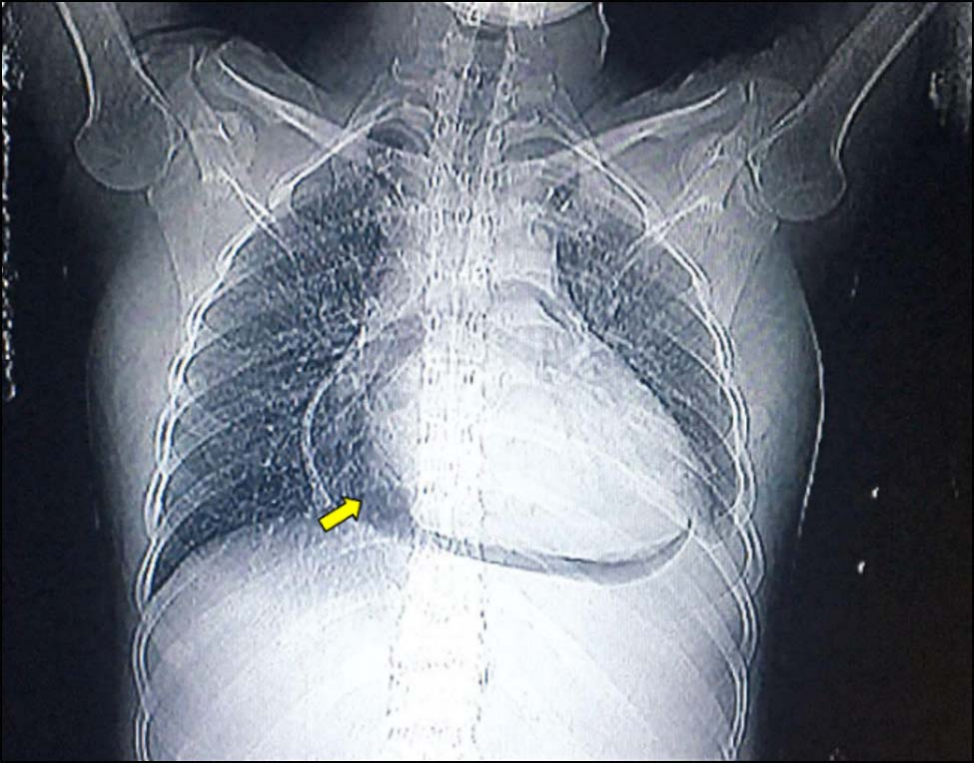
Chest X-ray showing pneumopericardium (yellow arrow).
